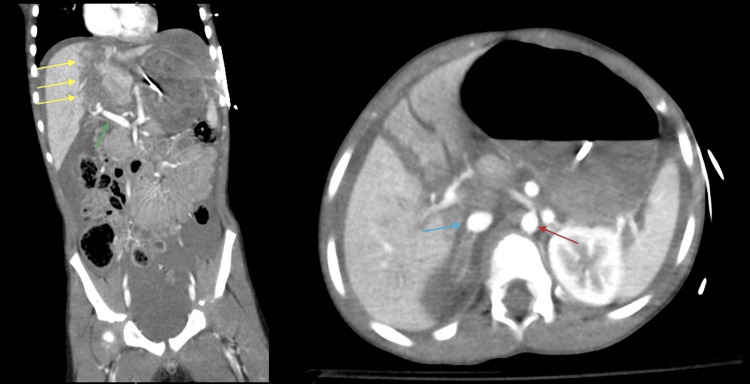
Computed tomography scan showing a high-grade liver injury Red arrow: aorta; blue arrow: inferior vena cava; green arrow: portal vein; yellow arrow: high-grade liver injury
